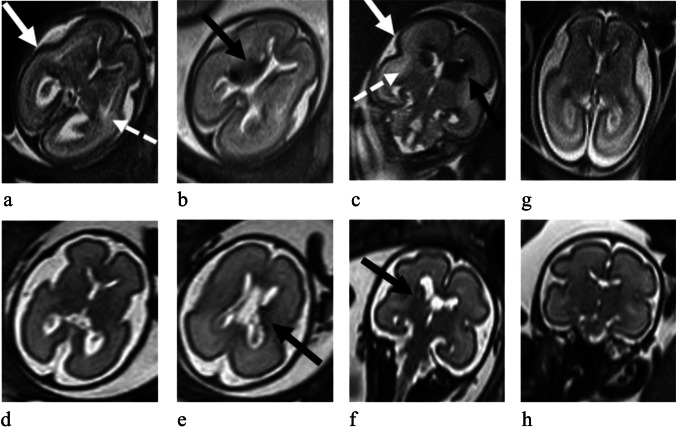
Axial (a and b) and coronal (c) ss-FSE T2-weighted sections from 22 week study of case ID07 showing in the anemic twin cortical cerebrospinal fluid (CSF) space reduction due to transient brain swelling (white arrows) and areas of high T2-weighted signal due to parenchymal edema (dotted arrows); black arrows point at germinal matrix hematoma associated with periventricular radial T2W hypointensities. g) unremarkable polycythemic co-twin for comparison. Axial (d and e) and coronal (f) from 28 week follow-up study of the anemic twin after laser treatment, depicting regression of brain swelling and of parenchymal edema areas, while periventricular sequelae of intraventricular haemorrhage (IVH) are present (black arrows). h) polycythemic co-twin follow-up is also depicted
